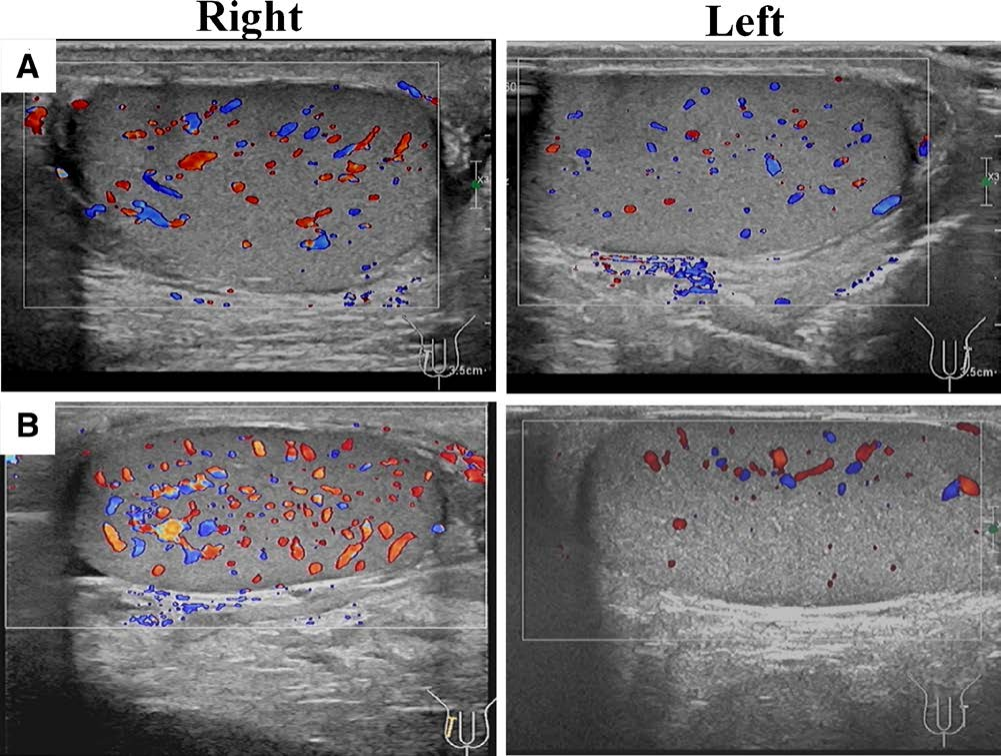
Transscrotal ultrasound imaging in a 34-yr-old patient with acute scrotal pain. (A) Initial color Doppler examination demonstrates markedly increased blood flow in the right testis, with comparative normal blood flow signals observed in the left testis. (B) The next day, an ultrasound reexamination showed that the vascular congestion in the right testicle was significantly increased compared to the left testicle and the previous examination.
